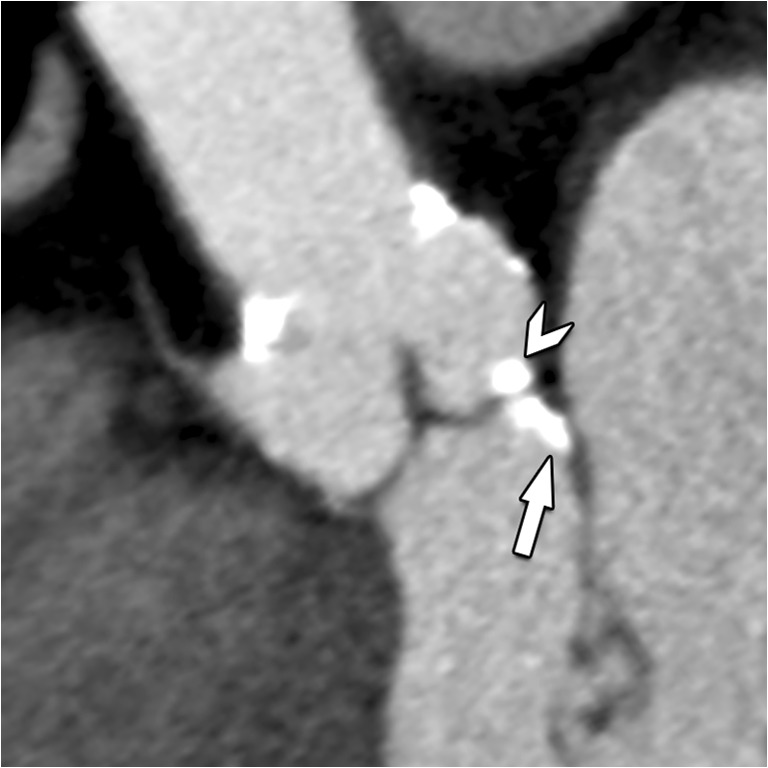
Double-oblique CT image of the aortic root in a TAVI candidate. While most calcification will be on a supra-annular level (arrowhead), occasionally, calcifications can also be found on an infra-annular subvalvular level (arrow). Reporting of these latter calcifications is important, as they can hamper proper deployment and attachment of the prothesis
